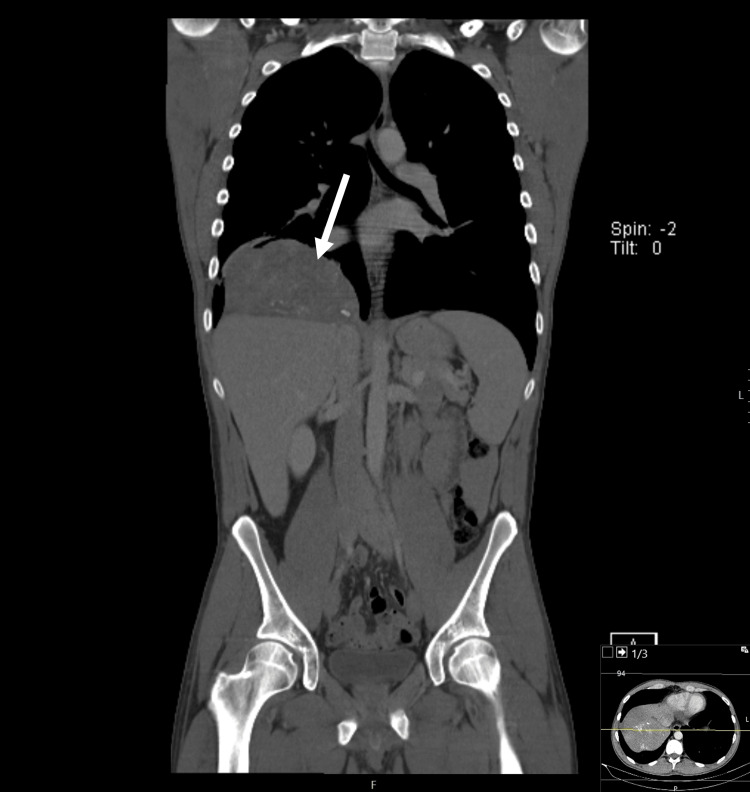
Chest, abdomen, and pelvic computed tomography (CT): coronal plane Hypodense mass, adjacent to the right part of the diaphragm and with extension into the thoracic cavity
